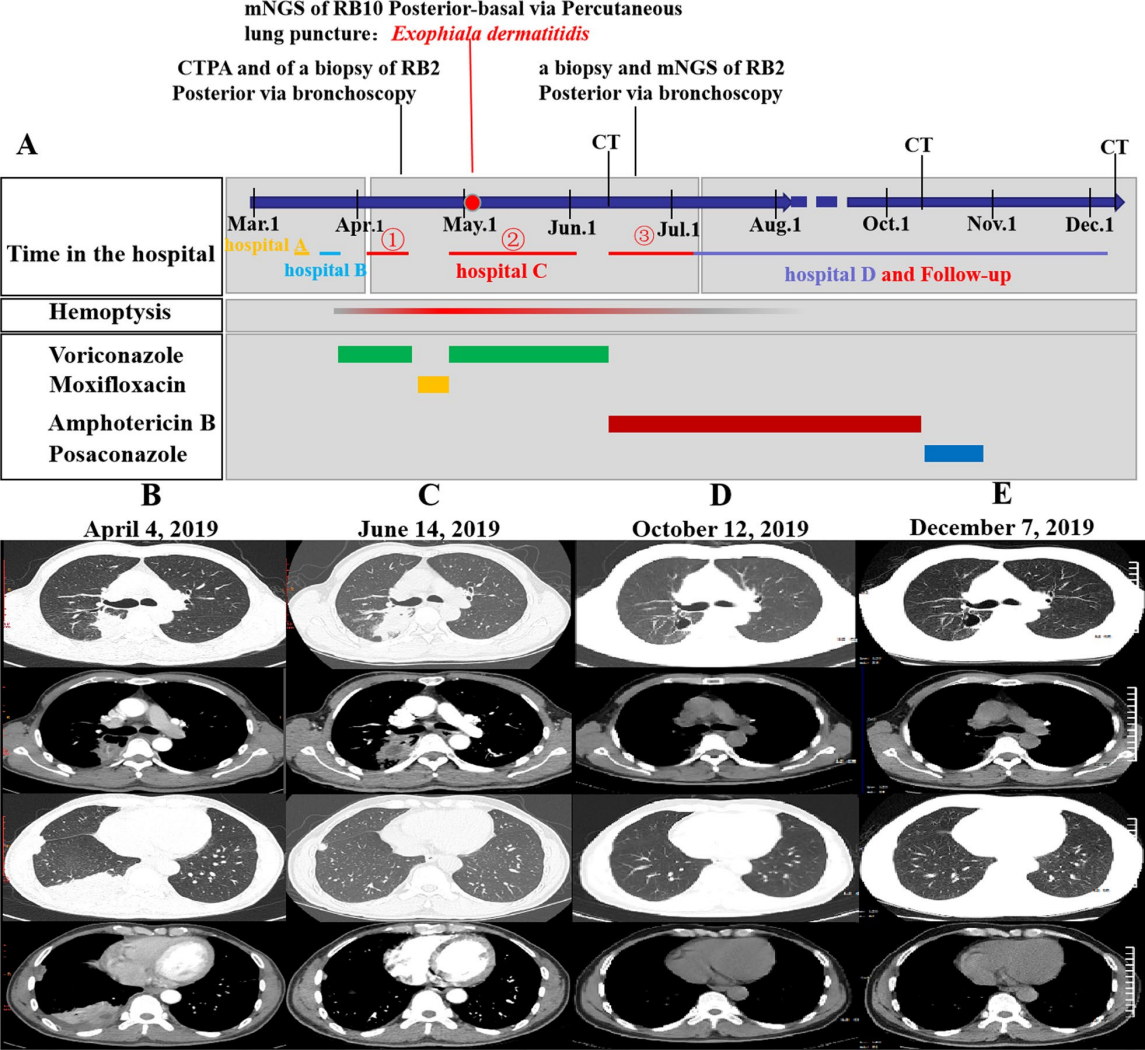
Timeline of clinical events and pharmacological treatments. The patient visited four hospitals successively; Hospital C was our hospital. He presented to our hospital three times and underwent three pathological biopsies and two mNGS tests. We performed follow-up chest CT scans and adjusted the medication accordingly. Hemoptysis resolved after treatment with amphotericin B. A Results of the first chest CT performed in our hospital (2019-4-11). Multiple nodules, mass lesions, and patchy shadows were present throughout both lungs, mainly under the pleura and particularly in the right upper and lower lobes. The results also showed unclear borders, an internal cavity, and moderate enhancement, with a standardized uptake value (SUV) of approximately 34/60/71 µ. B Results of chest CT re-examination after two months (2019-6-14). Some lesions in the posterior right upper and right lower lobes progressed, while others had been obviously absorbed. C Results of chest CT after 3 months of amphotericin B treatment (2019-10-12). The lesions had been obviously absorbed. D Results of follow-up chest CT after drug withdrawal for more than two months (2019-12-7). No change was observed compared to the last imaging findings
